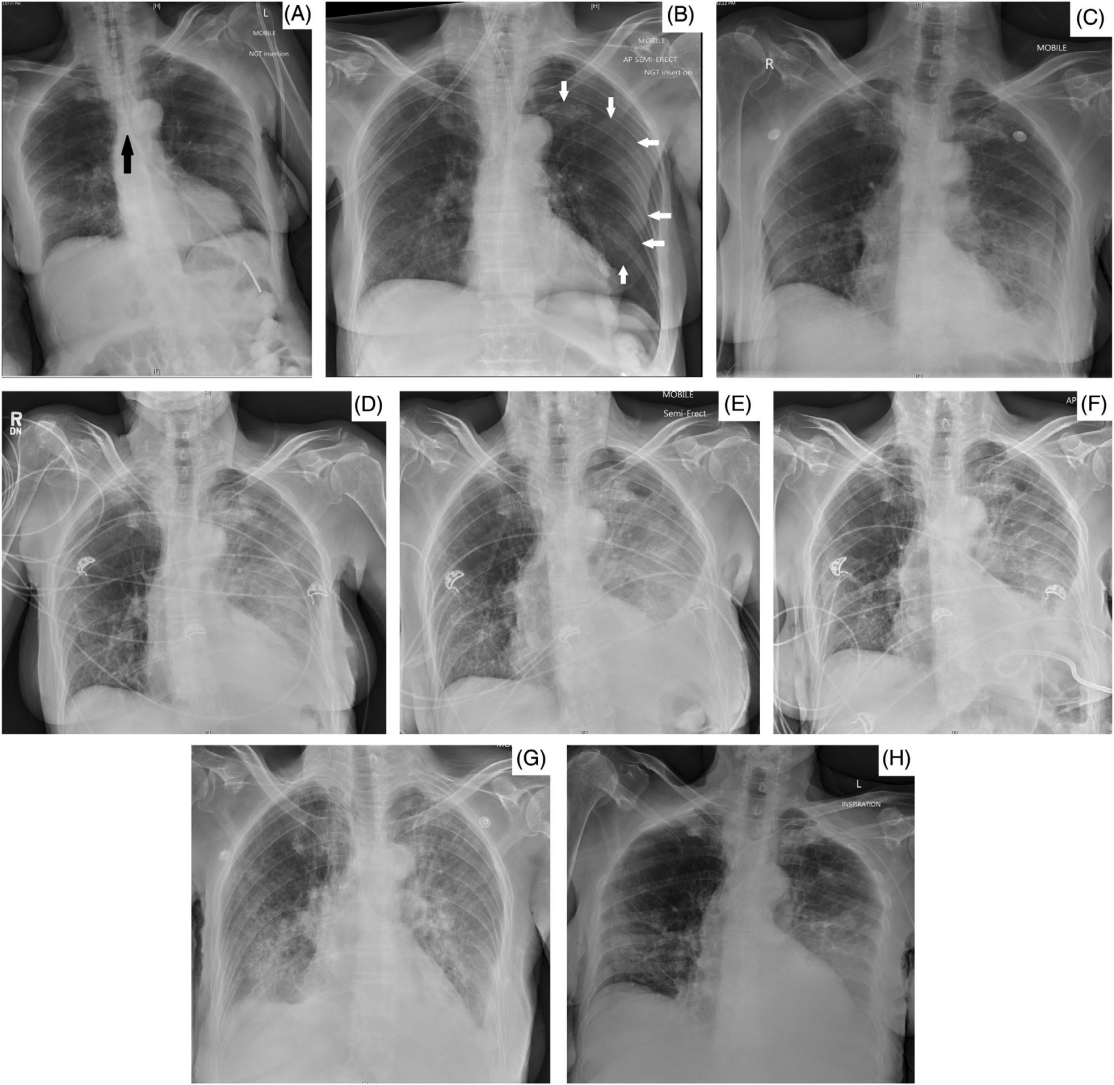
Chest X‐rays showing 1st NGT insertion through left main bronchus—black arrow denotes the sudden deviation of the NGT to the left indicating pulmonary misplacement into the left main bronchus (A), pneumothorax following 1st NGT insertion—white arrows delineating the pneumothorax after removal of the NGT (B) and serial chest X‐rays showing progression of empyema following instillation of NG feeds after the 2nd complicated NGT insertion (C–H). (D) Day 1 after removal of NGT following pleural NG feed instillation and CT identification of NGT in the lung base abutting the hemidiaphragm. (E) Day 2. (F) Day 3. (G) Day 3, following intercostal chest drain insertion. (H) Day 8, following intercostal chest drain removal after drainage has been maximized. (I) Day 15, showing improvement of empyema. There are persistent bilateral lung infiltrates from persistent aspiration secondary to oropharyngeal dysphagia
